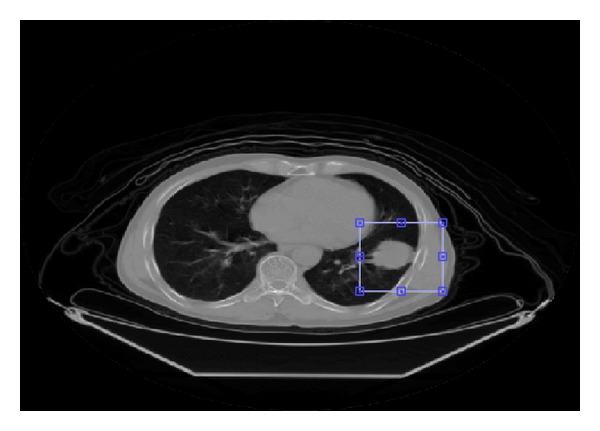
An axial CT slice of a patient with the region to be segmented marked.
